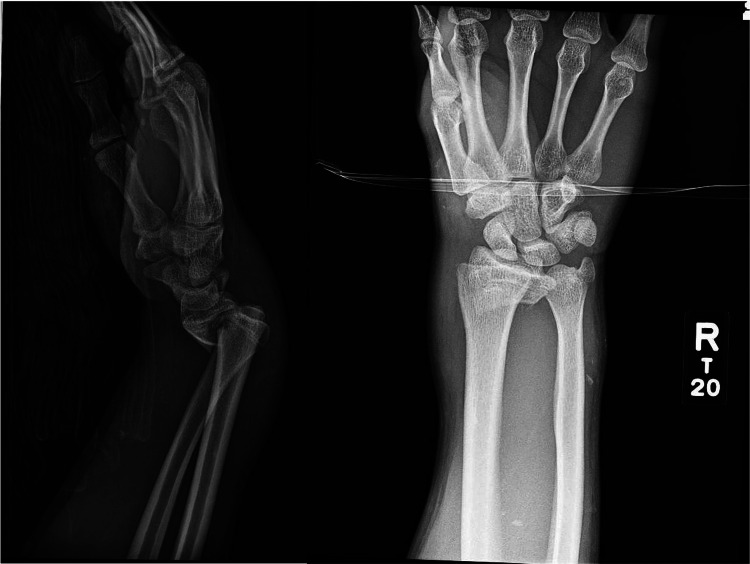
Radiographs at presentation Radiographs demonstrating the volarly displaced Salter-Harris type 4 distal radius fracture.
